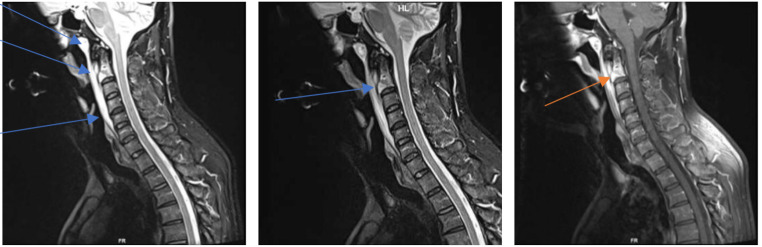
Contrast-enhanced MRI scan showing bone marrow edema of the base of the dens axis with adjacent soft tissue swelling including the soft tissue of the tonsils
